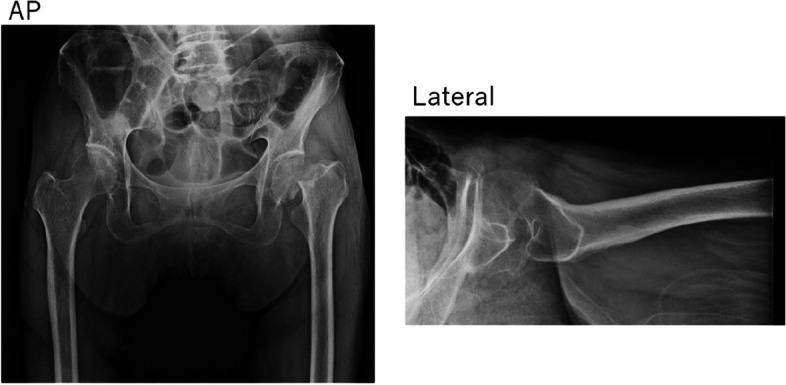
Preoperative hip plain X-rays of a representative case. (Garden classification Stage 4). Case. 81-year-old female diagnosed with a femoral neck fracture. Ambulatory status before the injury was Level 1
